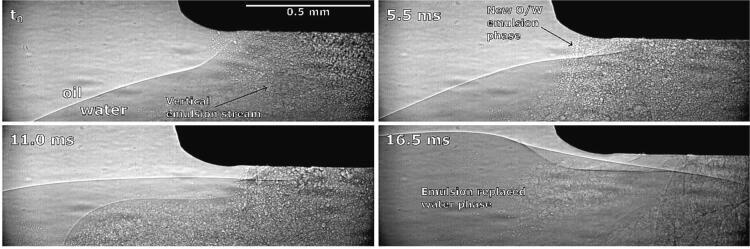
Synchrotron observation of vertical emulsion stream and oil–water interface movement around ultrasonic horn.
